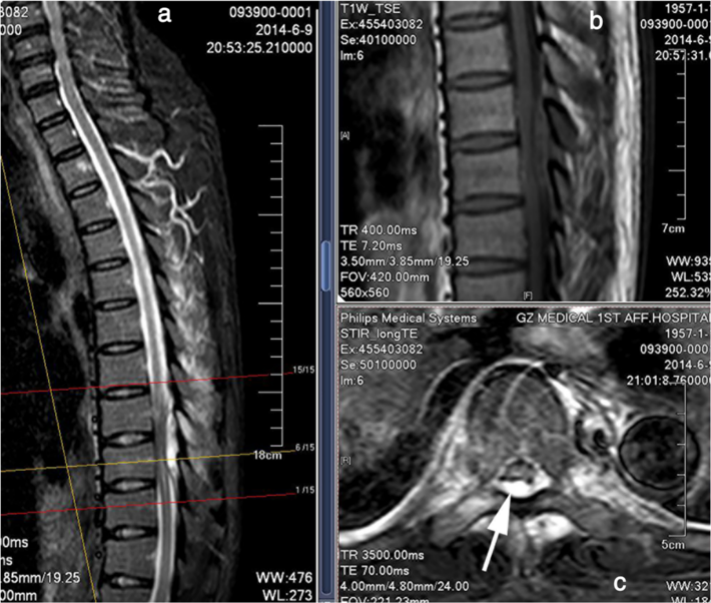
Magnetic resonance imaging of the thoracic vertebra. a Contrast-enhanced T2-weighted sagittal scan demonstrates a high-signal lesion behind the dural sac at the T8–9 level. b T1-weighted sagittal scan shows that the lesion has an area of moderate signal intensity and low-signal spots. c T2-weighted axial scan shows a high-signal area in both the vertebral canal and paravertebral muscle. The epidural abscess (single-headed arrow) is behand the spinal cord and dural sac
